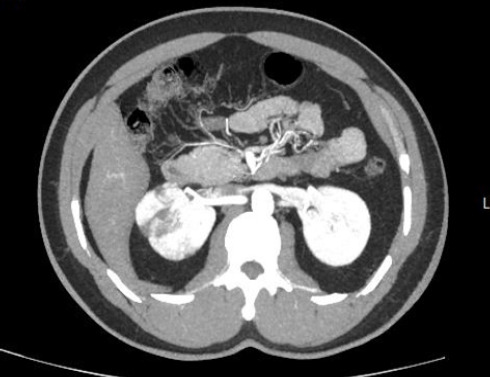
CT angiography revealed a wedge-shaped perfusion defect in the right kidney, consistent with renal infarction.
